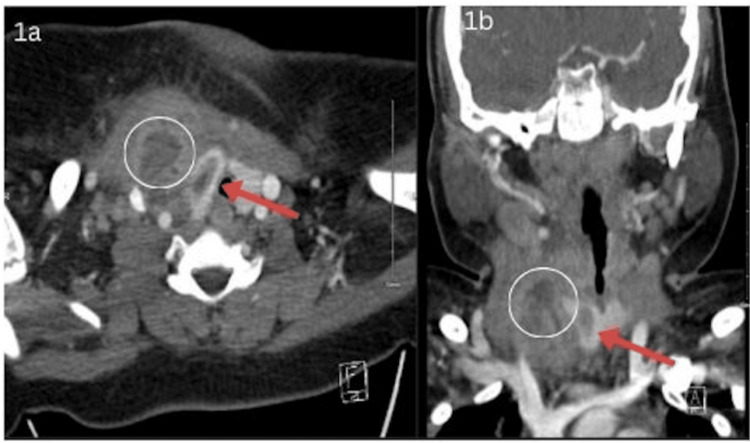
CT scan with contrast showing a 4 cm deep right neck abscess (circle) with an intrathyroidal component (red arrow) consistent with a type IV branchial cleft anomaly.
